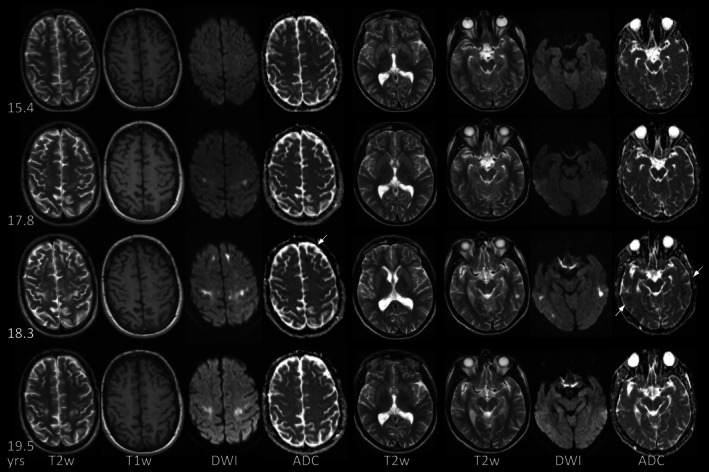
Exemplary white matter changes in p11. WM is normal in p11 at first MRI at 15.4 years. By 18 years, new, subcortical T2‐hyperintensity has developed in the bilateral precentral gyrus. At 18.3 years there is increasing involvement of frontal WM and new involvement of temporal WM with T1‐hypointensity and restricted diffusion (arrows). At 19.5 years during acute metabolic decompensation WM changes have decreased.
